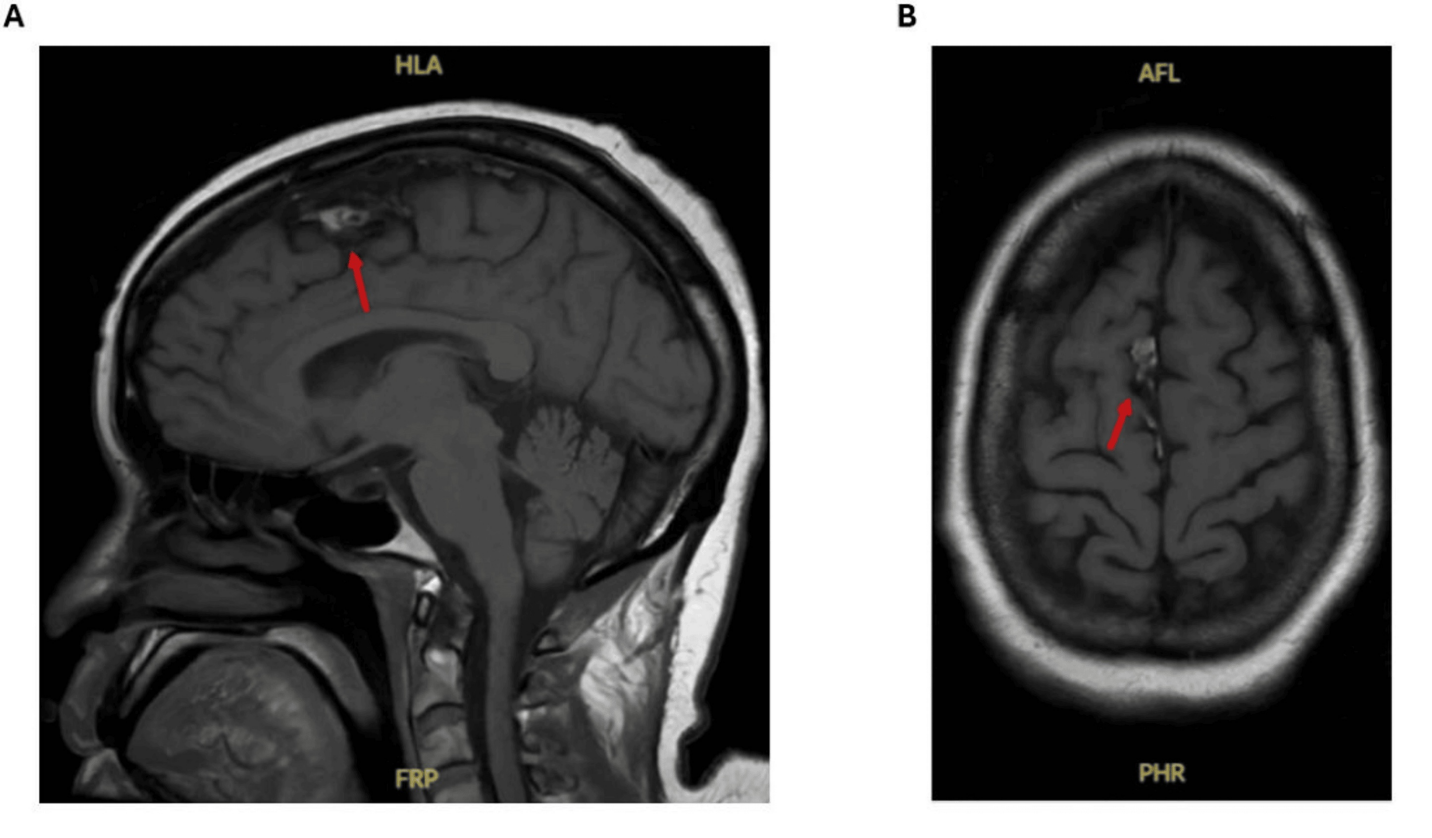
Sagittal (A) and Axial (B) MRI Sequences Showing a Punctate Acute Infarct in the Right Precentral Gyrus
